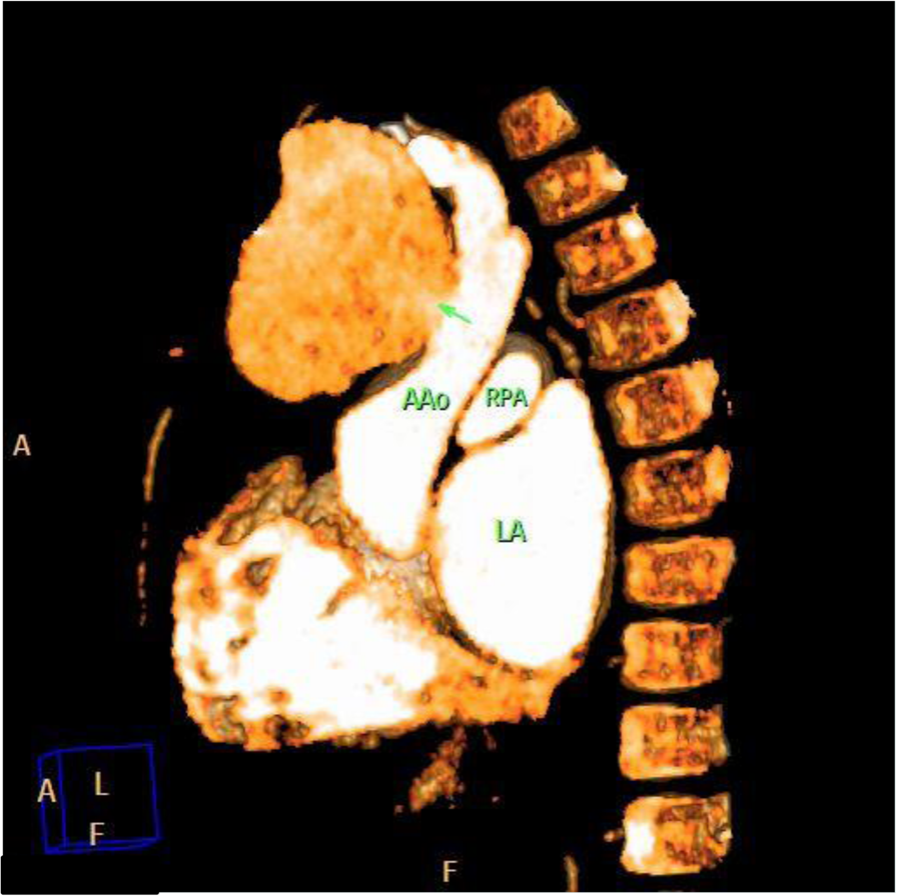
Computed tomography angiogram (colored) which shows a pseudoaneurysm arising from the anterior wall of the ascending aorta (arrow). Dimensions were 4.5 × 4.0 × 3.5 cm with a 10–11 mm neck. The aneurysm extended to the right and superiorly causing rightward deviation of the superior vena cava and innominate vein. *AAo* ascending aorta, *LA* left atrium, *RPA* right pulmonary artery
